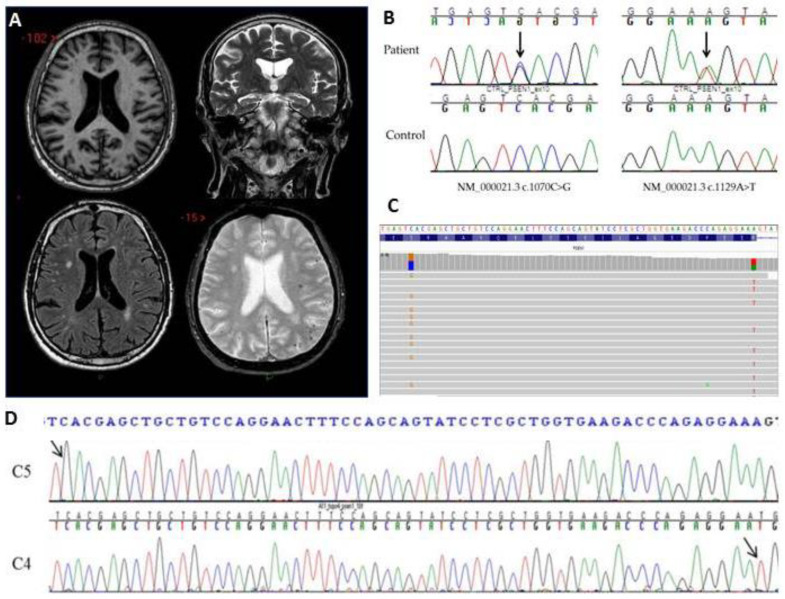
(A) Brain MRI: Axial T1 weighted image (upper left) shows a slight enlargement of the fronto-parietal sulci and of the posterior sylvian fissures; Coronal T2-weighted image (upper right) shows initial diffuse brain atrophy (temporal horns width with Schelten’s Medial temporal lobe atrophy (MTA) score scale scored as 2 [10]; Axial FLAIR (lower left) shows multiple multifocal asymmetric white matter lesions, mainly involving the deep and subcortical bi-hemispheric white matter; Axial T2-GE image (lower right) shows punctate multifocal hypointensity located hemorrhages, located mainly in the cortico-subcortical junction in the left hemisphere; (B) Electropherogram showing the heterozygous variant c.1070C > G in the exon 10 of *PSEN1* in the patient compared to a control sequence and the electropherogram showing the heterozygous variant c.1129A > T in the exon 10 of *PSEN1* in the patient compared to a control sequence; (C) IGV visual inspection of the two variants. Reads that read the c.1070C > G variant (orange) do not read the c.1129A > T variant (red) and vice versa; (D) Electropherograms from two different clones: the clone C5 carries only the c.1070C > G (arrow) variant while the clone C4 carries only the c.1129A > G variant (arrow).
